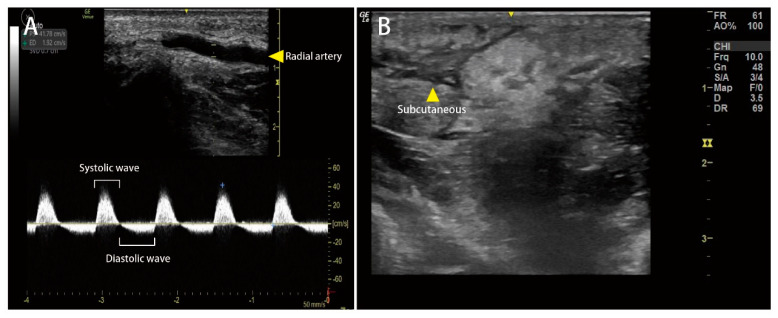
Point-of-care ultrasound (POCUS) findings in a patient bitten by *P. mucrosquamatus,* obtained one hour after the snakebite. (A) Diastolic retrograde arterial flow (DRAF) was noted in the affected limb, indicating that the target artery was compressed. (B) Cobblestone-like appearance (yellow arrowhead) located in the subcutaneous area.
